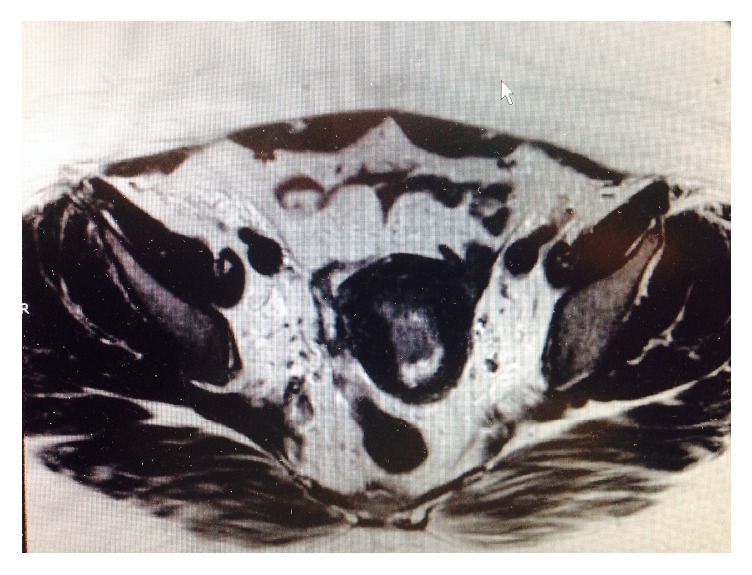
Magnetic resonance imaging of pelvic organs with Gadavist intravenous contrast demonstrated a 3.5 × 1.2 × 1.5 cm T2 enhancing mass with less than 50% invasion into the myometrium. No evidence of local regional metastatic disease.
